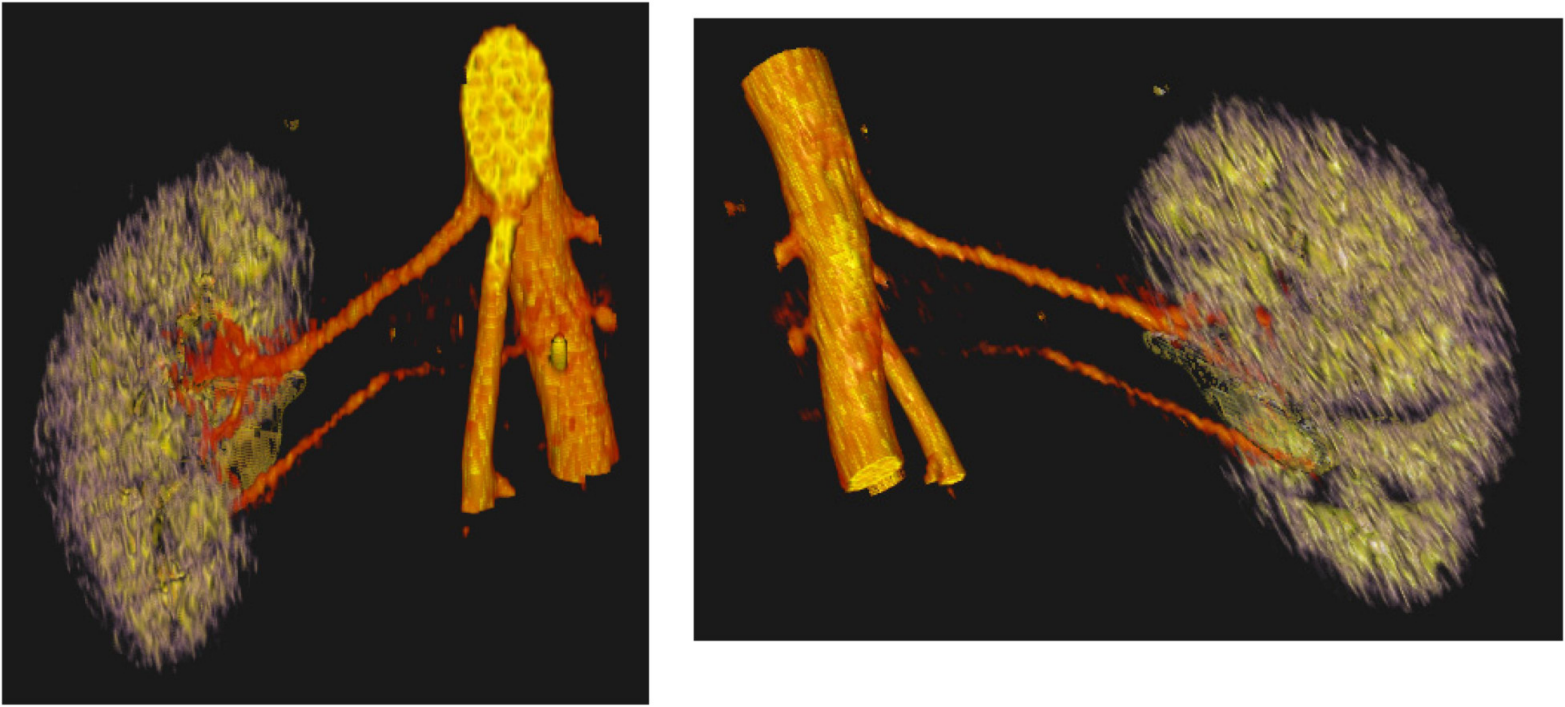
Patient A: ventral (left) and dorsal (right) views of the kidney showing a caudal renal artery that was not reported in the initial CT assessment.
